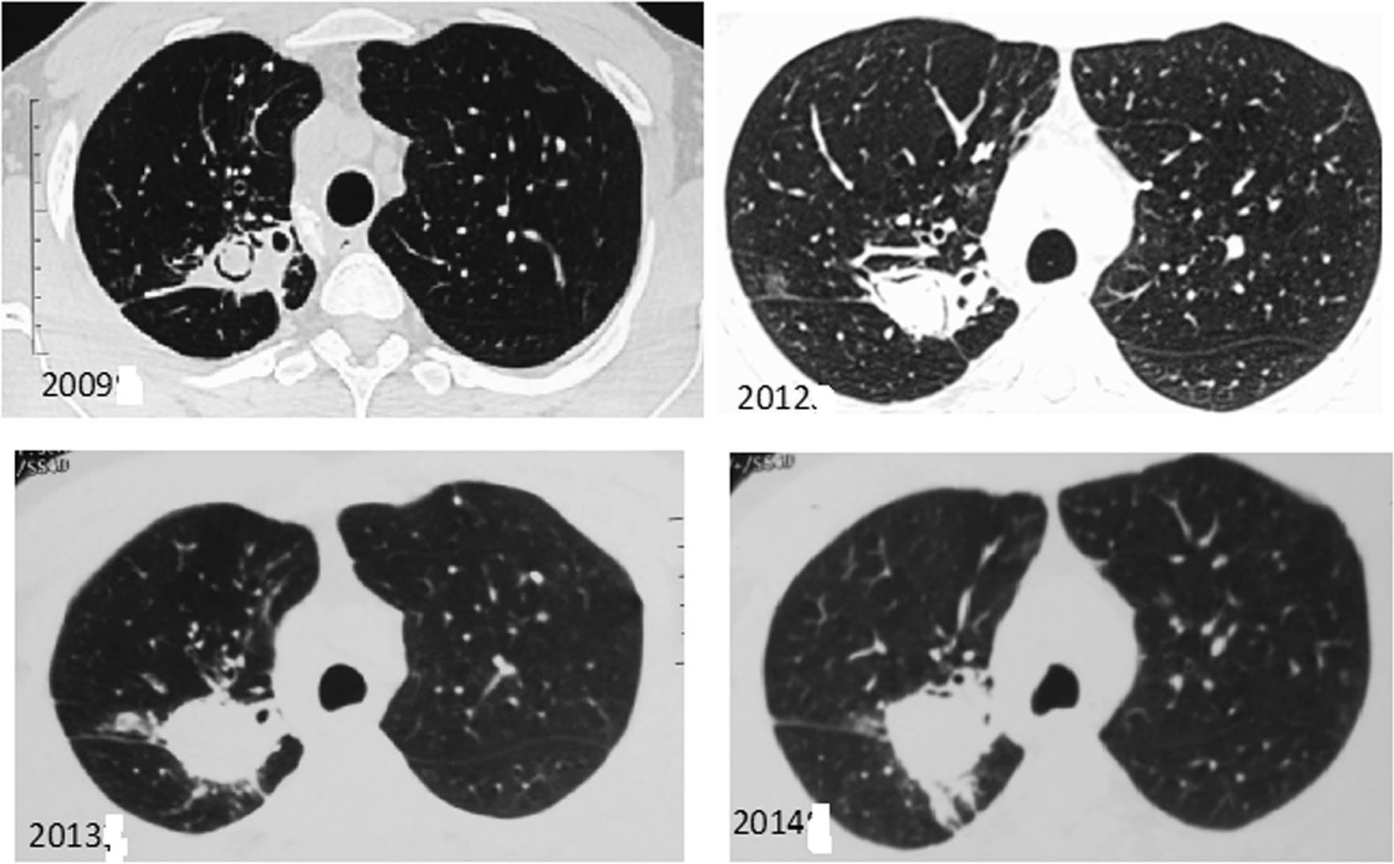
HRCT cuts from 2009 to 2014 showing development of aspergilloma in *right upper* lobe which grew over 5 years
